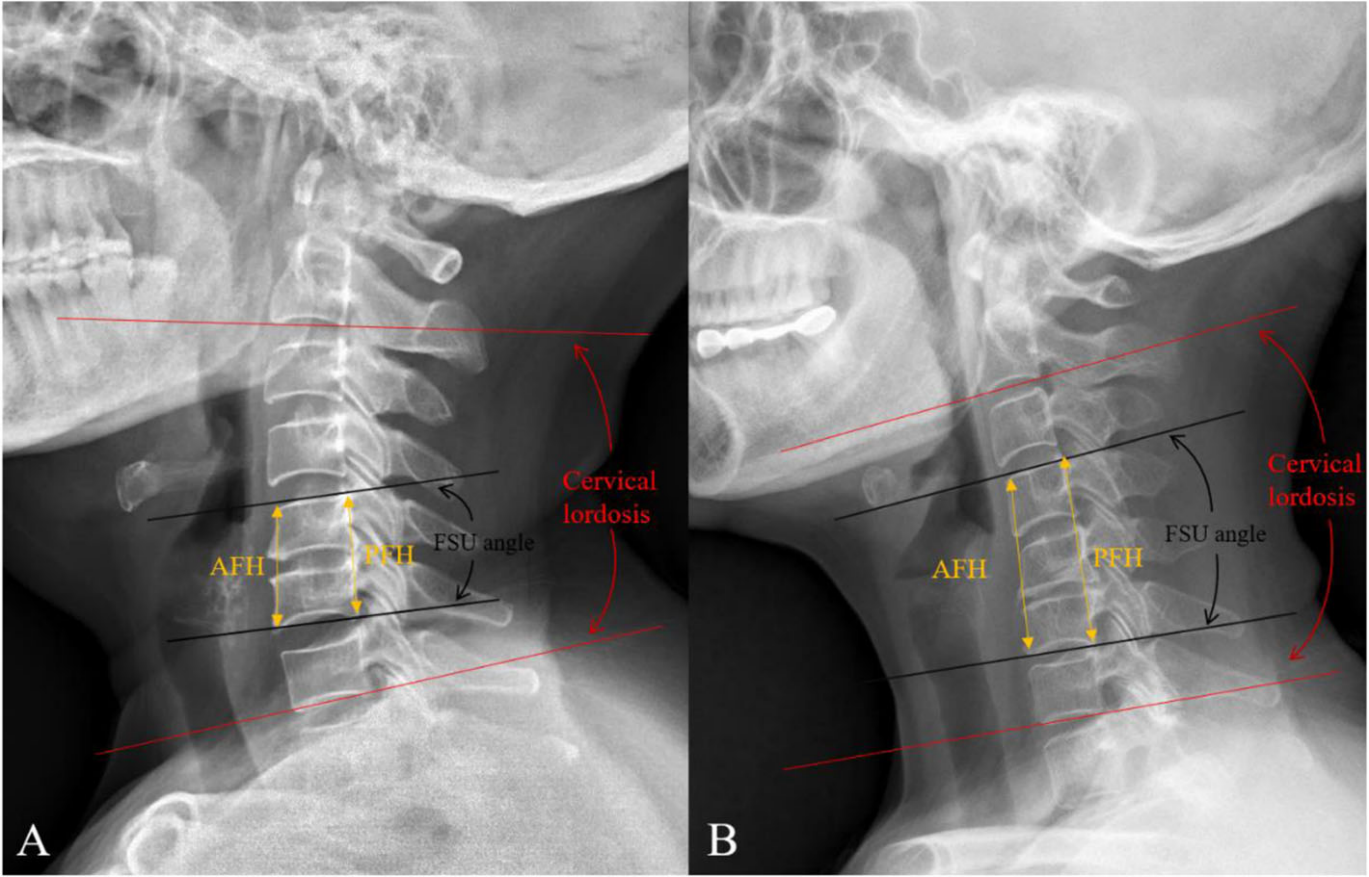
The measurement method for radiological parameters. The angle between the inferior margin of the C2 vertebrae and the inferior margin of the C7 vertebrae was measured as cervical lordosis, while the lines between the superior endplate of the cephalad vertebral body and inferior endplate of the caudal vertebral body were measured as the FSU angle. The FSU height was measured as the distance from the highest portion of the upper end plate of the cephalad vertebra to the lowest portion of the lower end plate of the caudal vertebra in the anterior or posterior FSU. The ROM was the difference in CL and FSU angle between the full flexion and extension radiographs. CL, cervical lordosis; FSU, functional spinal unit; AFH, anterior FSU height; PFH, posterior FSU height
